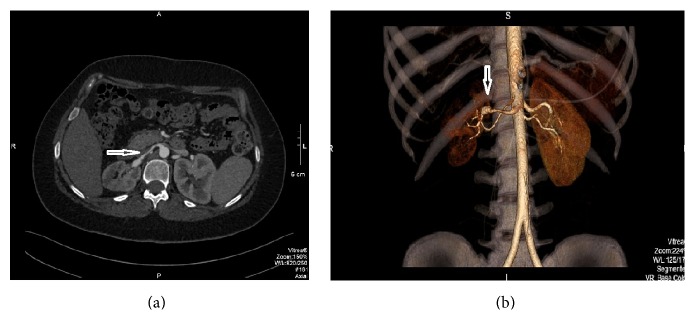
CT angiography of the renal arteries. Transverse view (a) and 3D rendering (b) showing the right renal artery (arrow).
